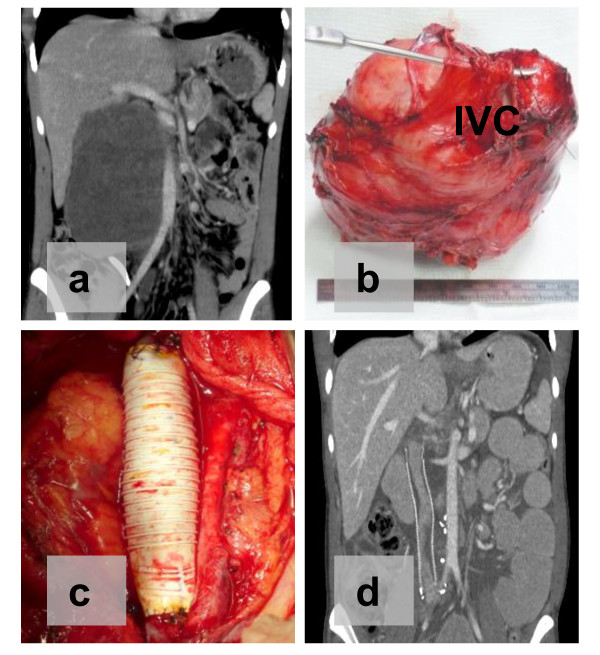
Retroperitoneal Tumor Involvement of the Inferior Vena Cava (IVC). Preoperative computed tomography scan demonstrates IVC involvement without obvious venous collateralization (a). The tumor was resected en bloc with a portion of the IVC (b) and reconstructed using synthetic polytetrafluoroethylene (PTFE) tube graft (c). Follow-up imaging was done postoperatively (d).
